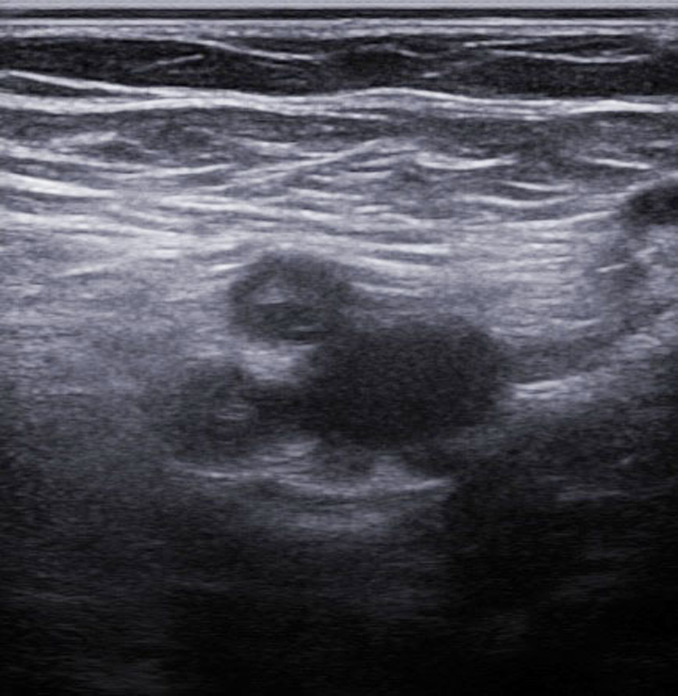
echo Doppler of left lower limb showing occlusion of femoral axes
